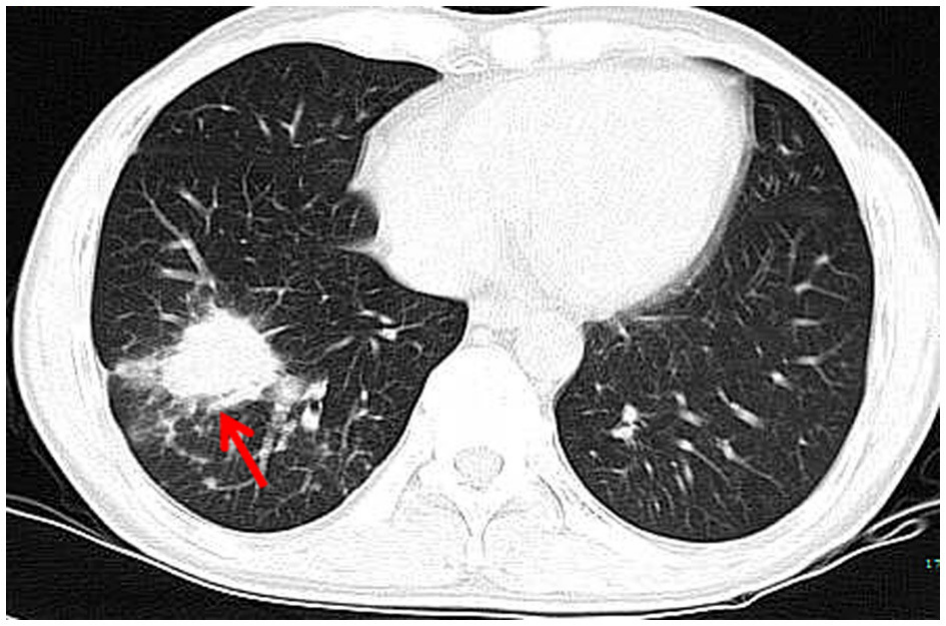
A chest CT scan revealing a mass measuring 3.7 × 3.0 cm in size in the right lower lung (red arrow).
